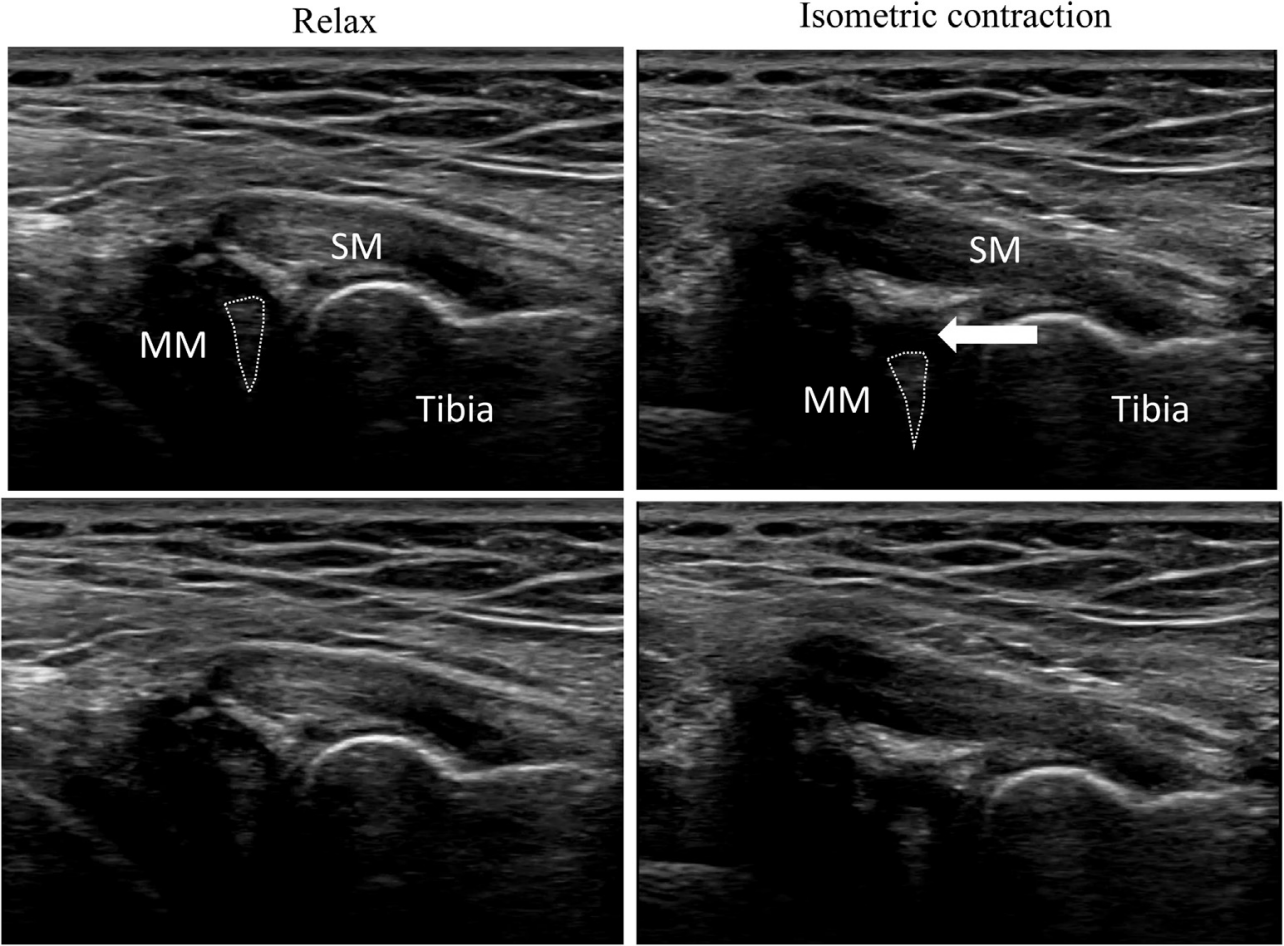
Long-axis ultrasound image at the knee joint line. The probe is placed at approximately 90° just above the semimembranosus tendon to obtain a long-axis view. Semimembranosus tendon located above the medial meniscus. The patient is instructed to perform isometric contractions in knee flexion with the support of an assistant. If there is a ramp lesion, a low echoic space appears below the semimembranosus tendon and joint capsule. The medial meniscus left behind the contraction of the semimembranosus muscle can be observed. The white arrow indicates the ramp lesion. Left is the relaxed state, and right is the isometric contraction state. (MM, medial meniscus; SM, semimembranosus.)
